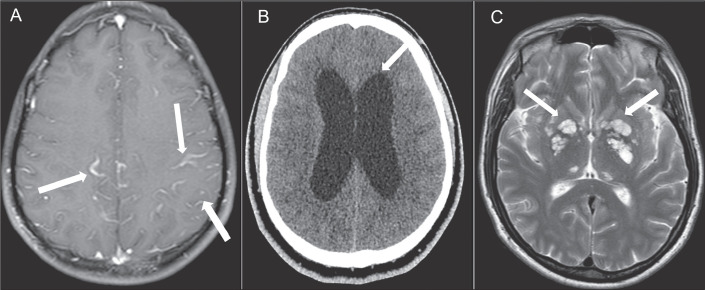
MRI scans of three different patients. A: Meningitis.
